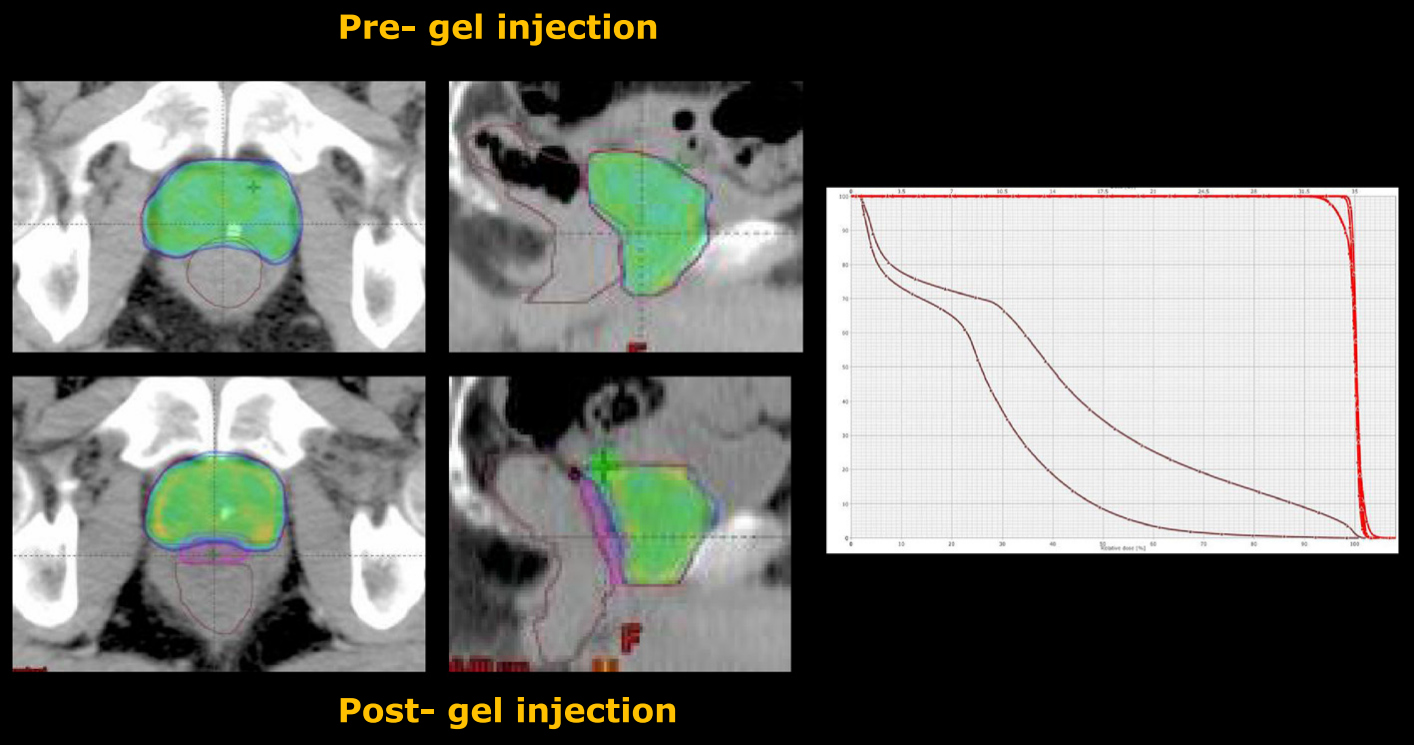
The potential dosimetric role of SpaceOAR™ gel implantation: dose distribution and DVH for a study patient where double CT scan and double planning was performed. Data are shown for CTV, PTV and rectum. No differences were observed for the target volumes while remarkable difference was observed in this case for the rectum.
